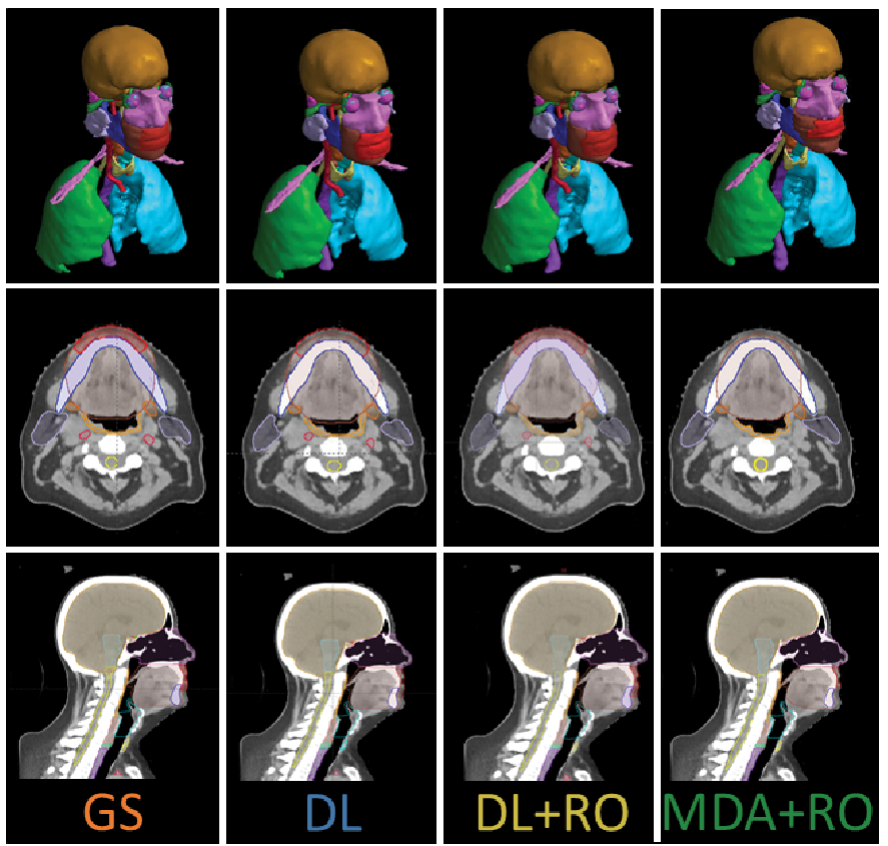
3D visualization, and an axial and sagittal slice of the CT with the contours from the gold standard (GS) dataset, as well as the DL, DL+RO, and MDA+RO contours for a representative patient.
